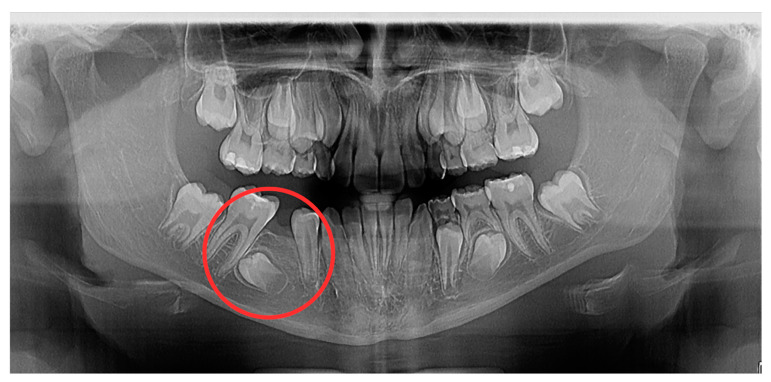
Panoramic radiograph showing the reduced space for the eruption of tooth 45 and the almost horizontal position of tooth 45.
